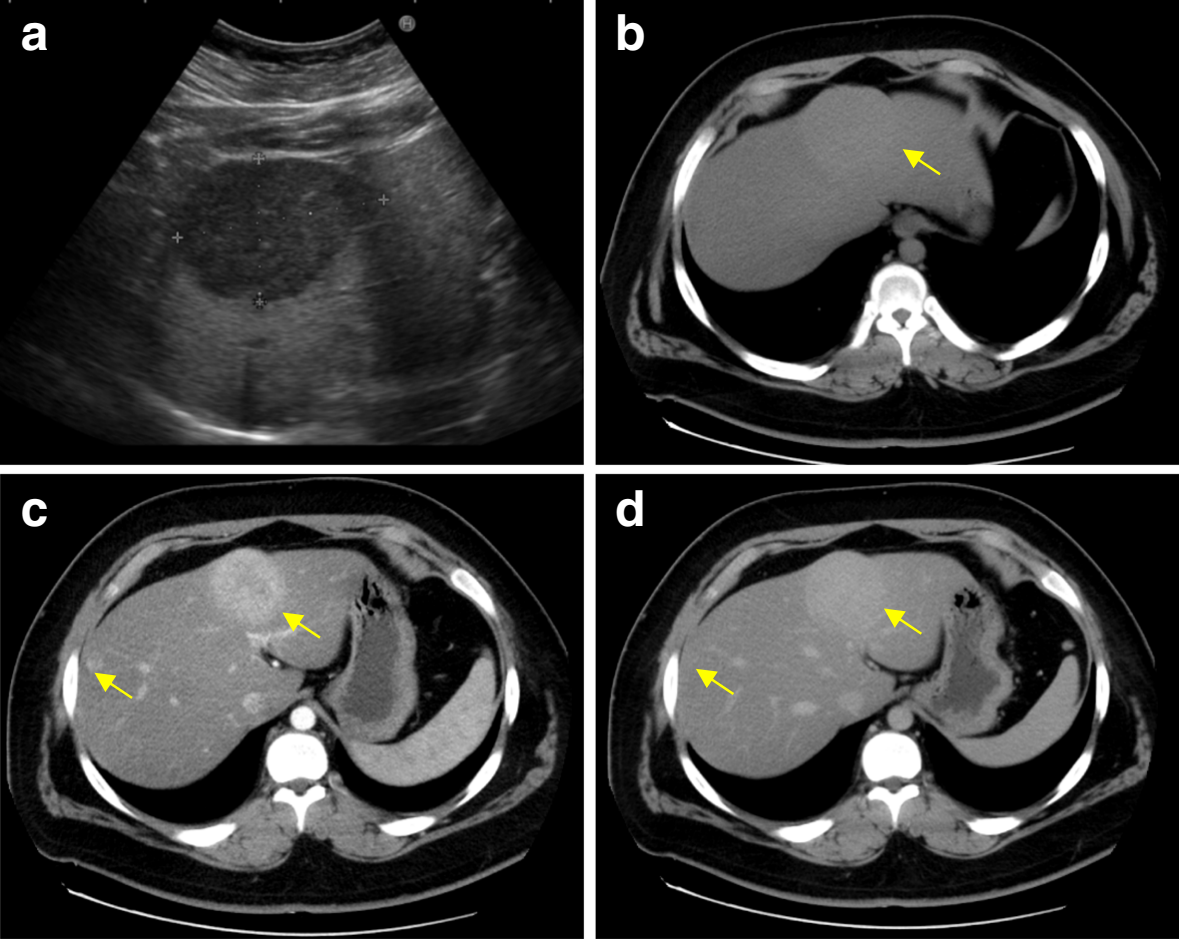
Preoperative ultrasonography and computed tomography images showing the presence of tumors. a Abdominal ultrasonography image showing a hypoechoic tumor approximately 7 cm in diameter protruding forward on the surface of the left lobe. b Plain computed tomography image showing an isodense tumor in the left lobe. c Contrast-enhanced computed tomography (CECT) image showing two tumors in segments 3/4 and 8 that were enhanced in the arterial phase. d CECT showing the two tumors with prolonged enhancement in the late phase
